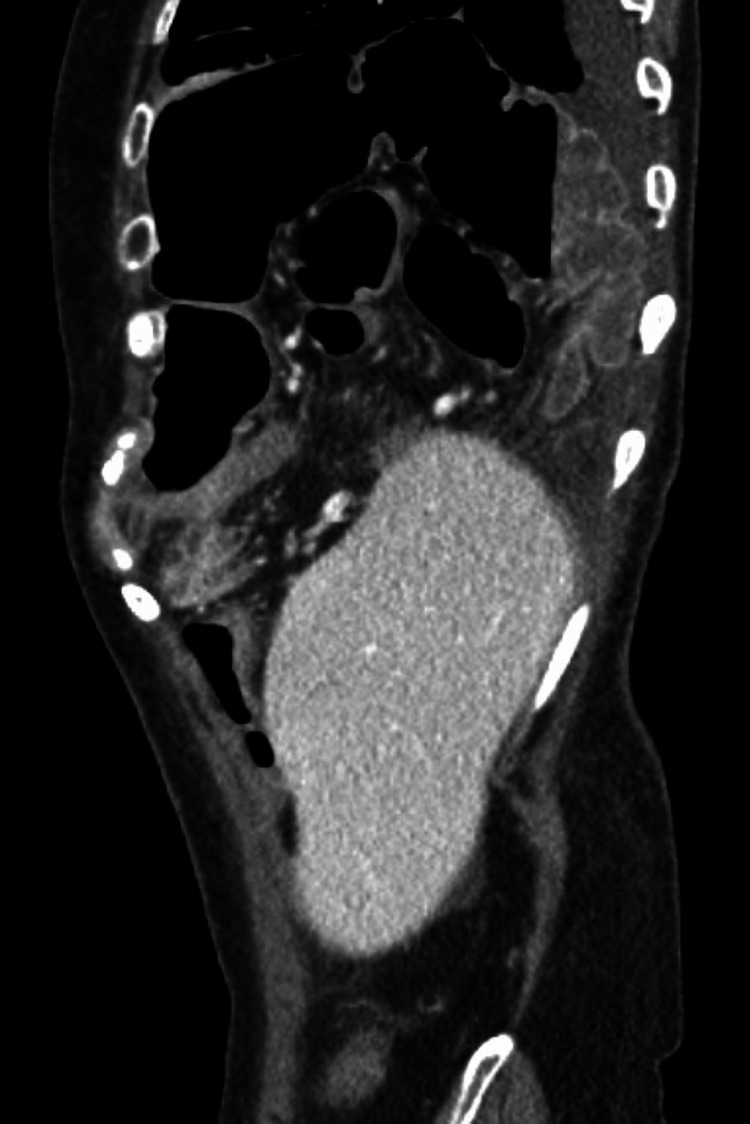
CT at presentation showing a right-sided, large diaphragmatic hernia containing portions of the ascending and transverse colon without evidence of obstruction, bowel wall thickening, or pneumatosis. Although the CT does not allow for the full examination of the right lung, it confirms total displacement and near-total collapse of the right lower lobe.
